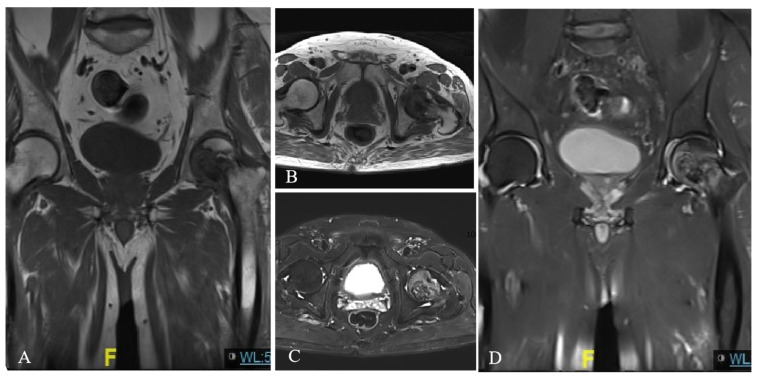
The coronal (A) and axial (B) T1-weighted images of bilateral hips showed a hypointense signal in the left femoral head, which showed the heterogeneous enhancement of the enhanced axial (C) and coronal (D) MR scans. “F” in Figure A and D represents "Front".
